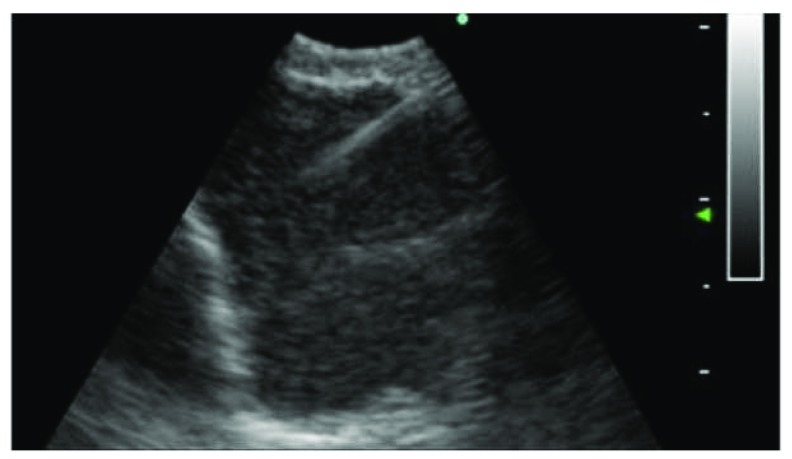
Lymph node sampled with a needle under ultrasound guidance. This figure is an original image taken in the author’s clinic for this publication.
